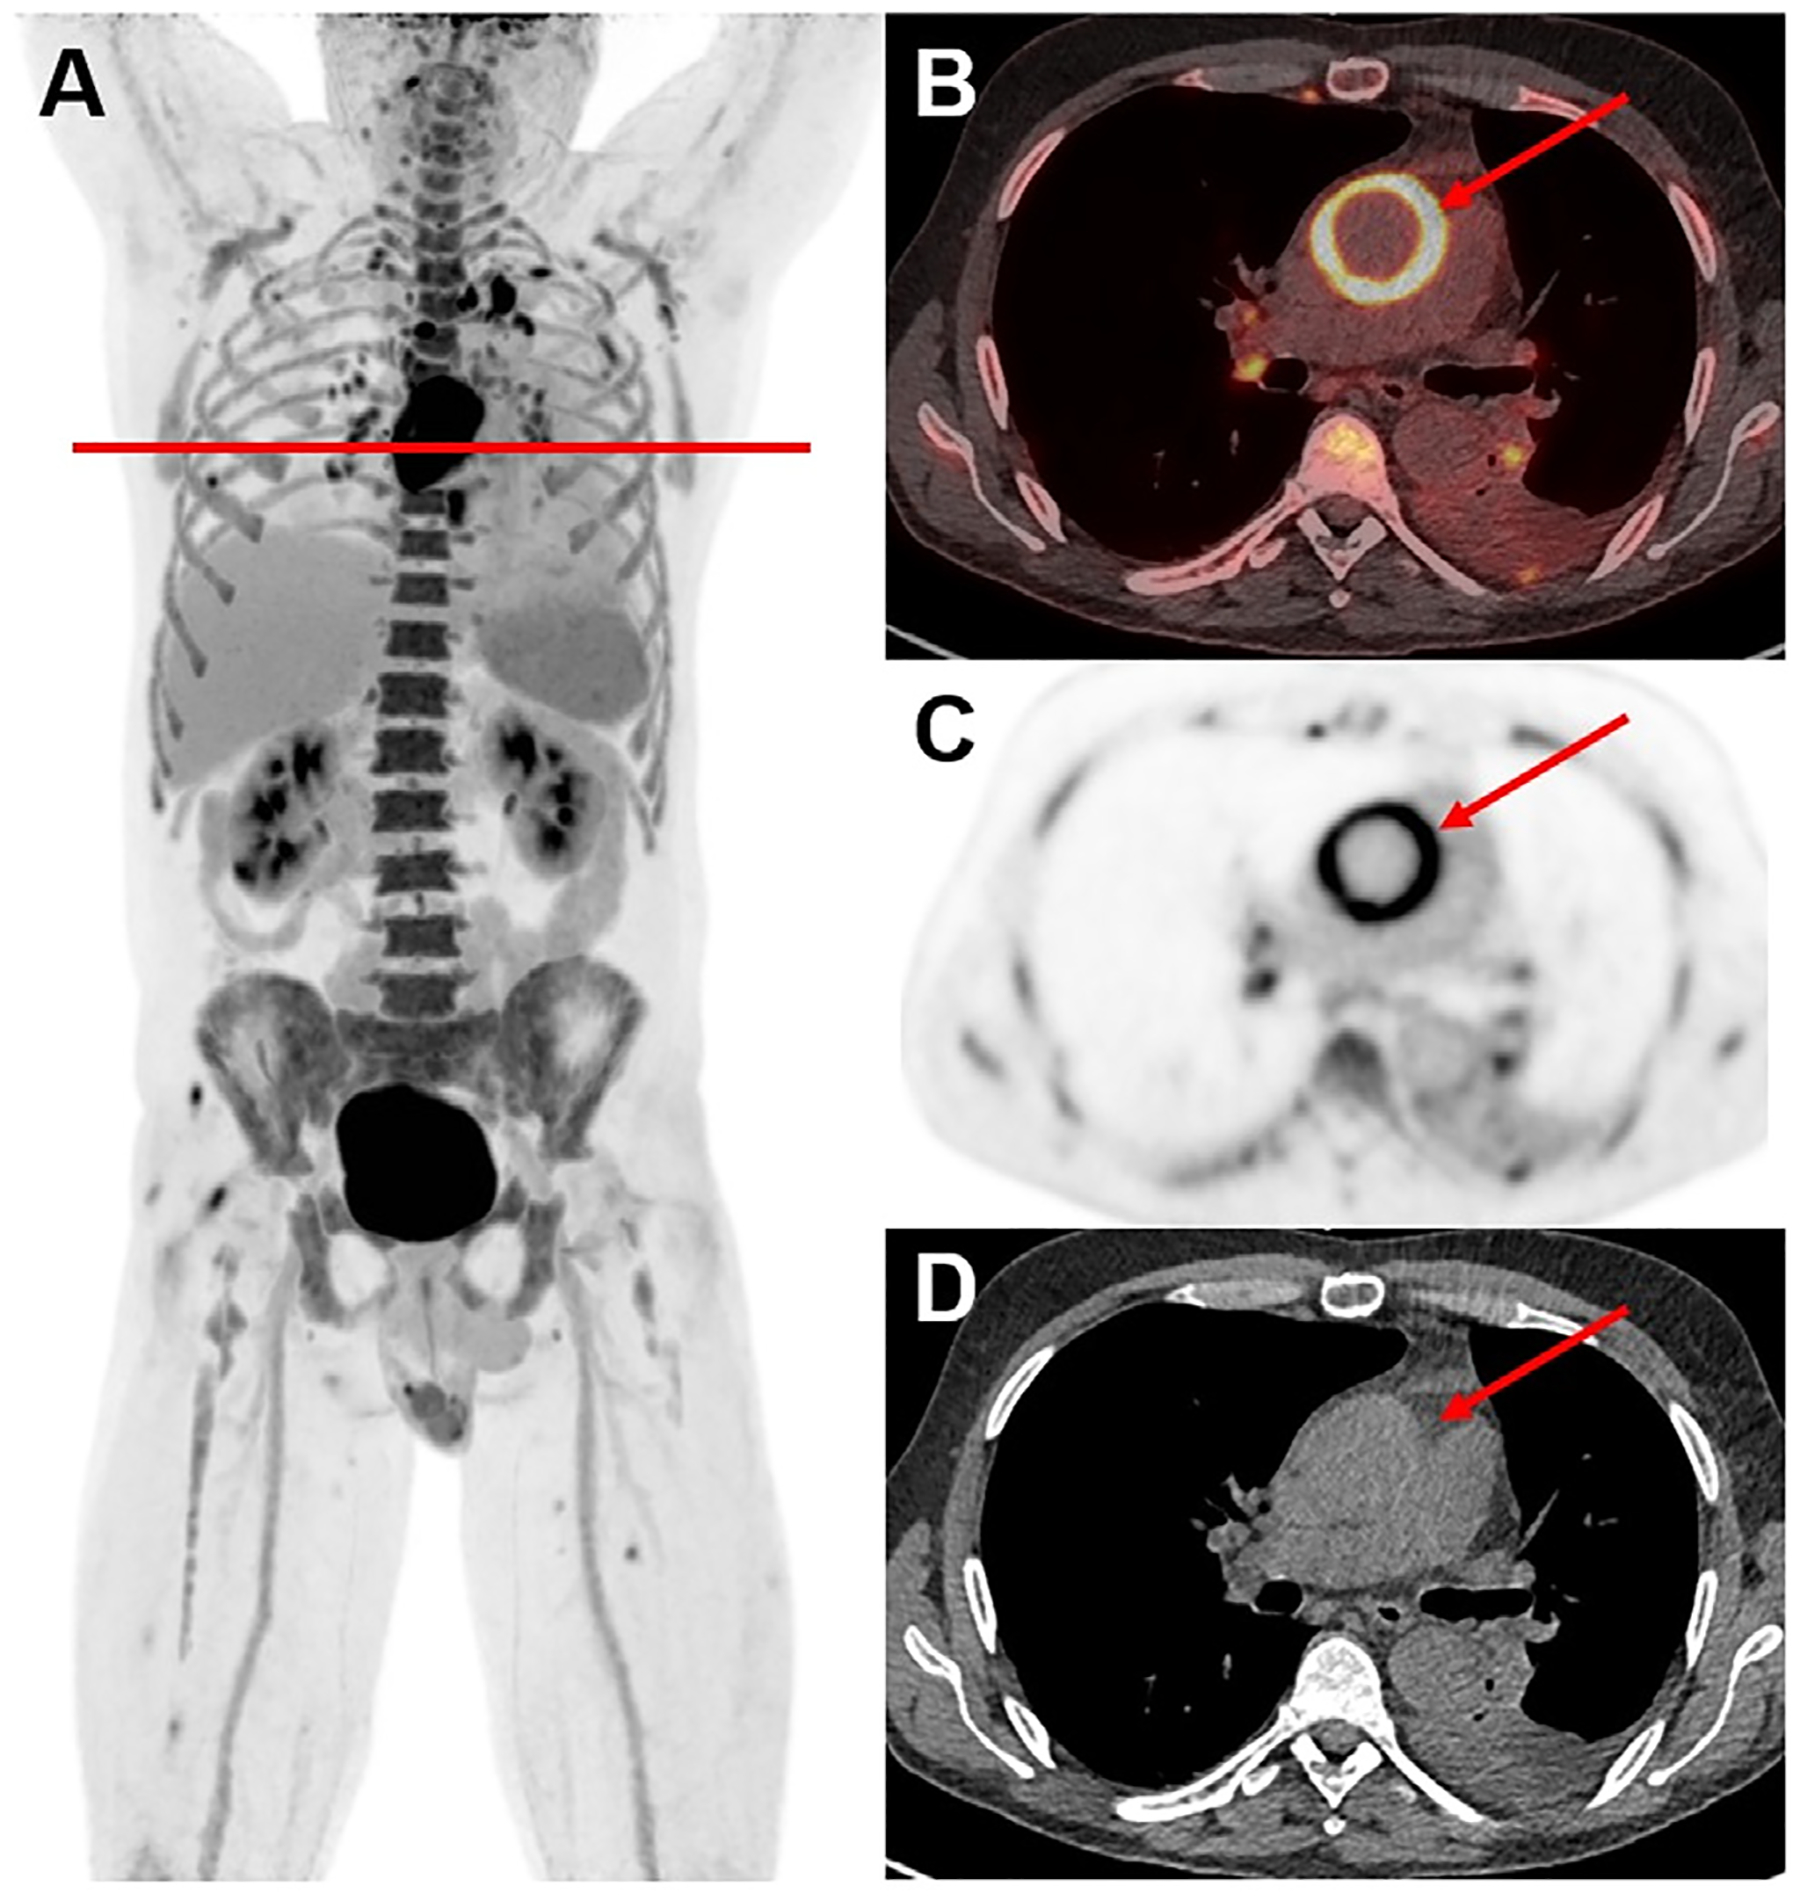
LAFOV PET/CT revealed a graft infection of the ascending aorta in a 59-year-old male patient. Shown are maximum intensity projection (A), LAFOV PET/CT (B), PET-only (C) and CT-only (D) images. The patient suffered from fever of unknown origin with positive blood cultures for streptococcus. LAFOV PET/CT not only revealed the main focus at the ascending aorta (red arrow), but also showed other infectious emboli in the lung and skeletal muscles.
